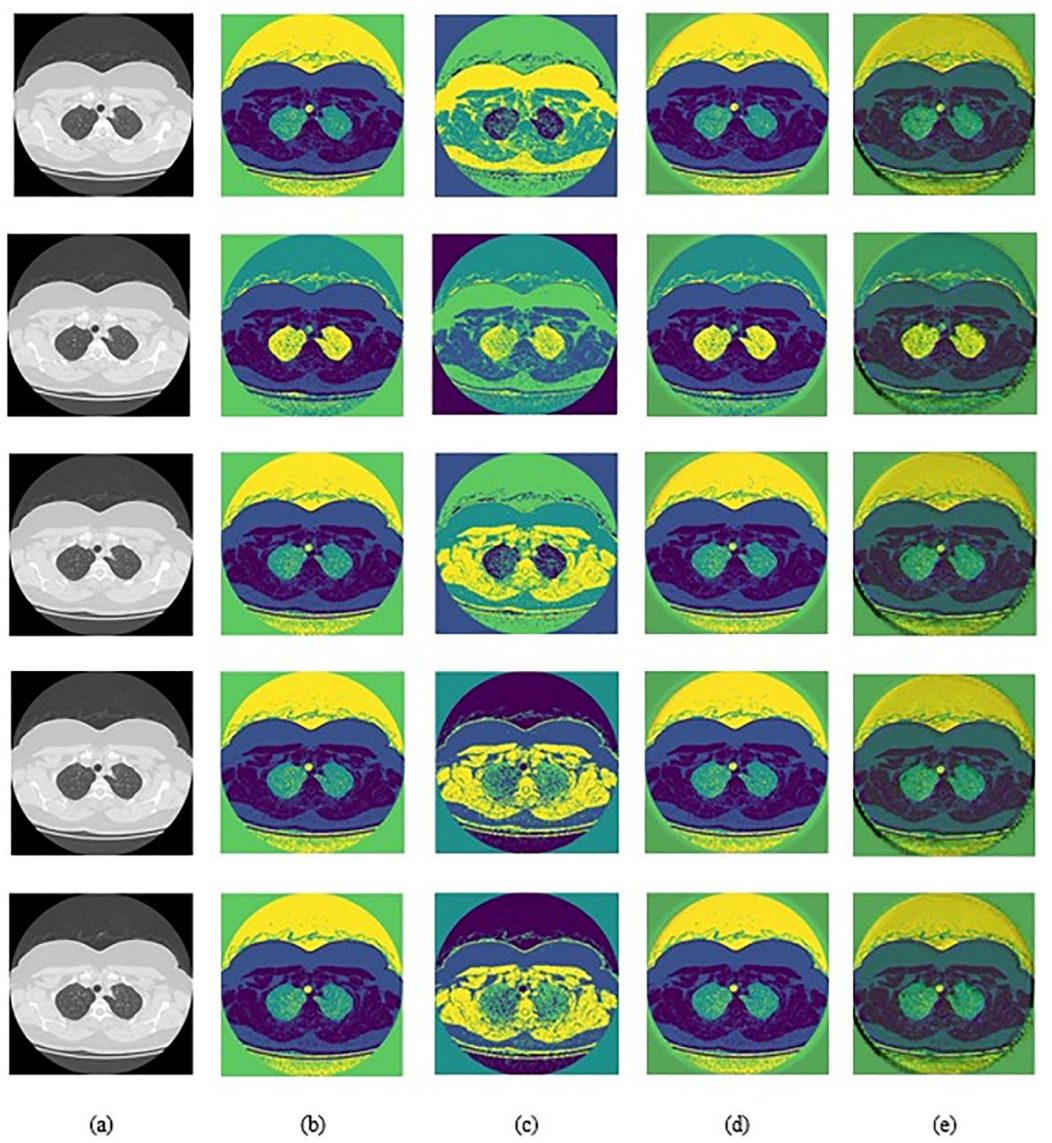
Image Results for Lung Disease Segmentation using CT images a) Input Image b) FCM c) nnU-Net d) K-Means e) Conventional SegNet and f) Modified SegNet.
